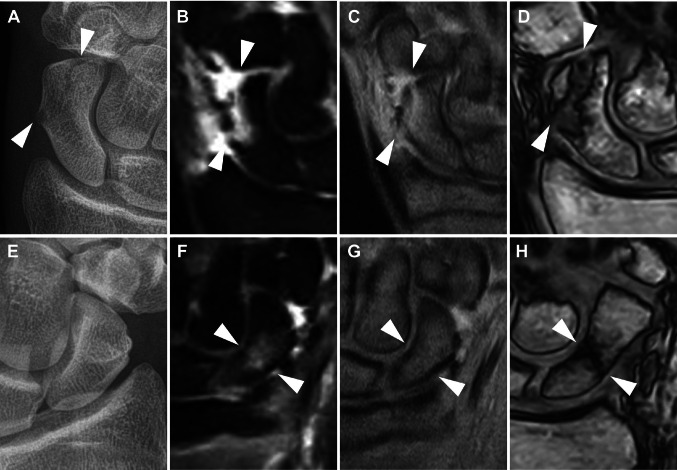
X-ray (A, E), standard MRI with coronal STIR (B, F) and coronal T1 in-phase (C, G), and the high-resolution 3D T1-weighted MRI sequence (D, H) of a stable scaphoid fracture of the tubercle type A1 in a 33-year-old female patient (A-D) and a non-displaced transverse fracture of the waist type A2 in a 28-year-old male patient (E–H). All fractures (indicated by arrowheads) are clearly detectable in the 3D T1-weighted sequence whilst the transverse fracture is not visible in X-ray (E) and T1 in-phase imaging (G) and with only edema in the STIR (F)
